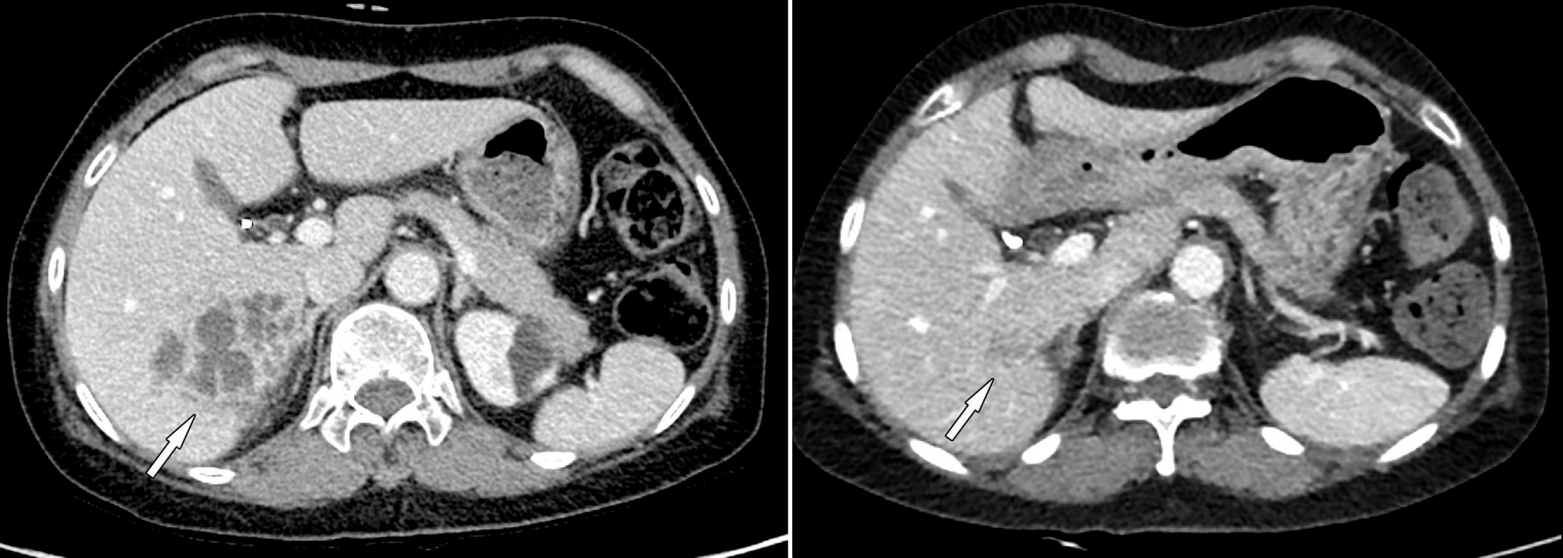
Contrast-enhanced CT images of a 61-year-old woman with liver abscesses. Body mass index was 20.4 kg/m^2^. (A) Initial standard-dose CT shows clustered peripheral enhancing lesions in the right posterior liver (arrow), suggestive of liver abscesses. (B) Follow-up ultralow-dose CT shows liver abscess nearly resolved (arrow). The CT dose index volume of initial CT and follow-up CT was 5.7 mGy and 1.8 mGy, respectively. Image quality and noise were comparable between initial CT and follow-up CT, and the diagnostic confidence of follow-up ultralow-dose CT was excellent. The scores for image quality, noise, and diagnostic confidence were 3, 3, and 5 for both readers.
